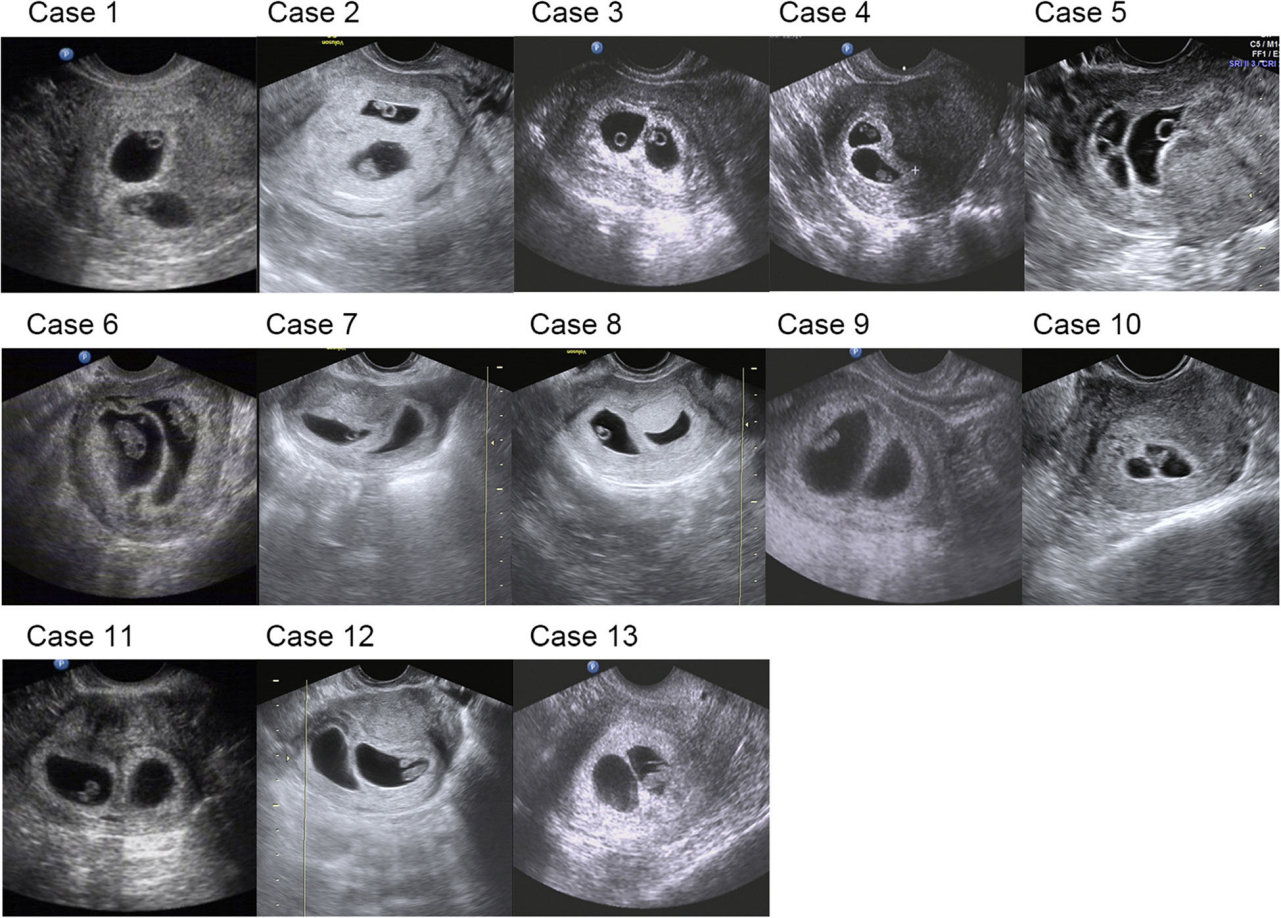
The images of the initial ultrasound at gestational 6–7 weeks. Case 1 to 6: gestational 6–7 weeks` ultrasound with 2 gestational sacs and 2 fetal poles. Case 7 to 13: gestational 6–7 weeks` ultrasound with 2 gestational sacs and 1 fetal pole
